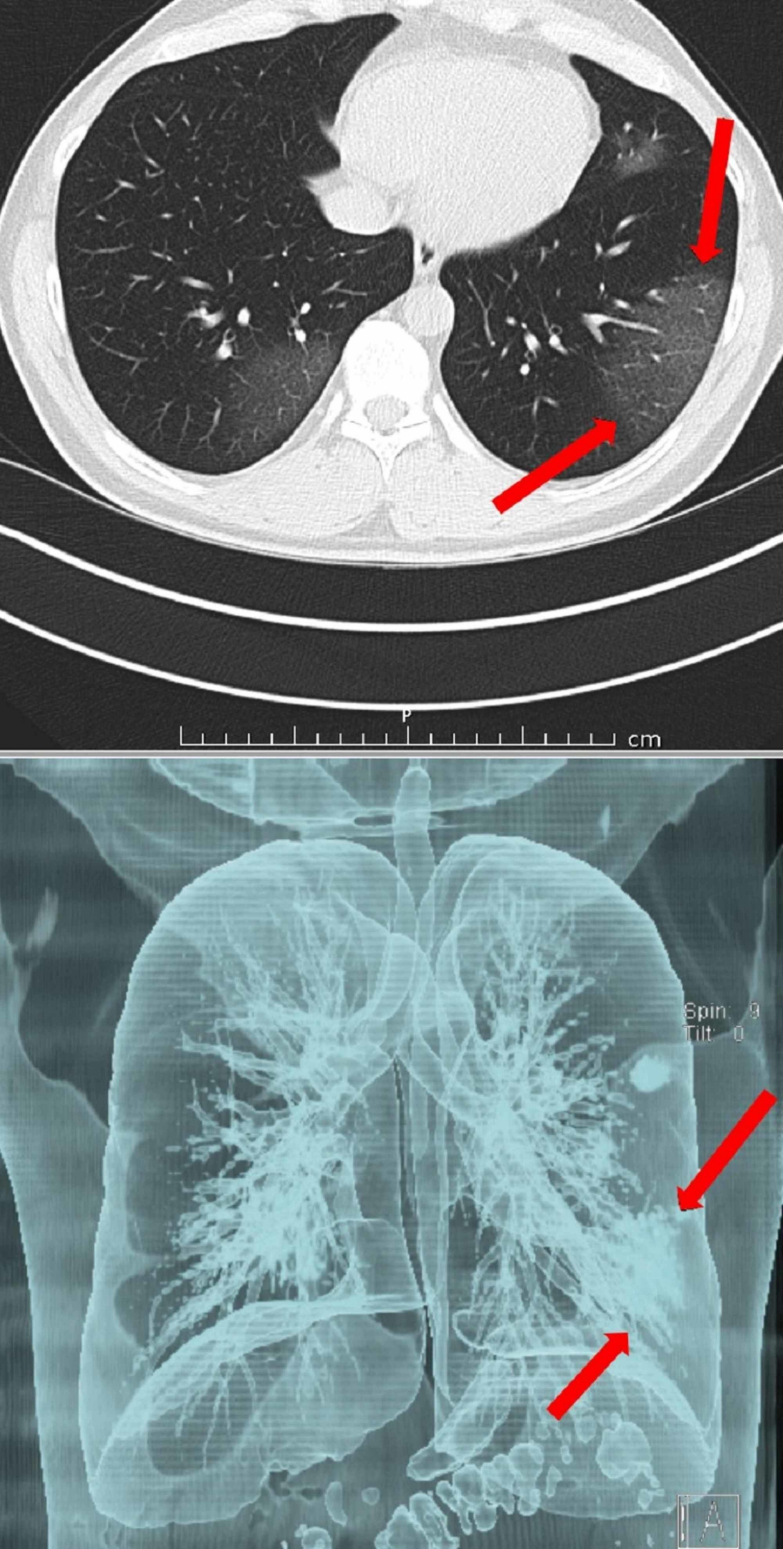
CT examination of the same patient 2 days later The upper left lesion is enlarged and the lower left lesion is visible. CT, computed tomography
